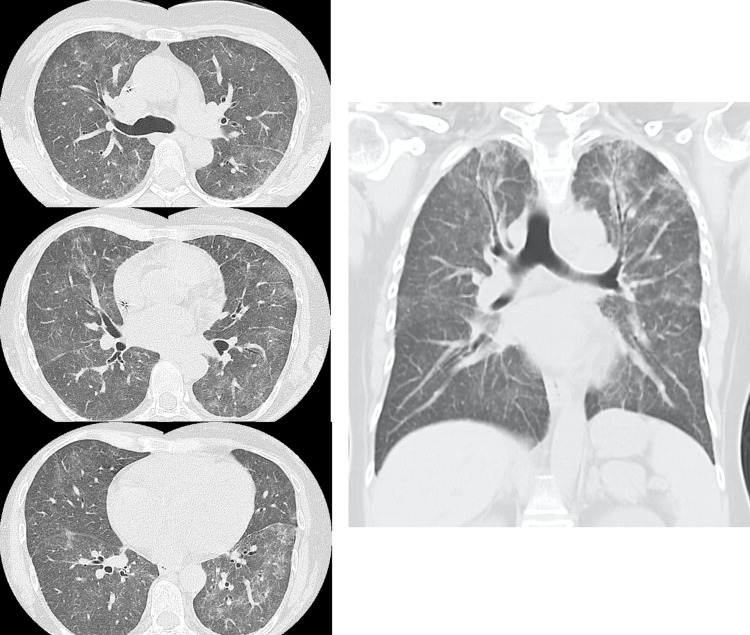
Chest CT images of the patient upon admission to Hamanomachi Hospital.
